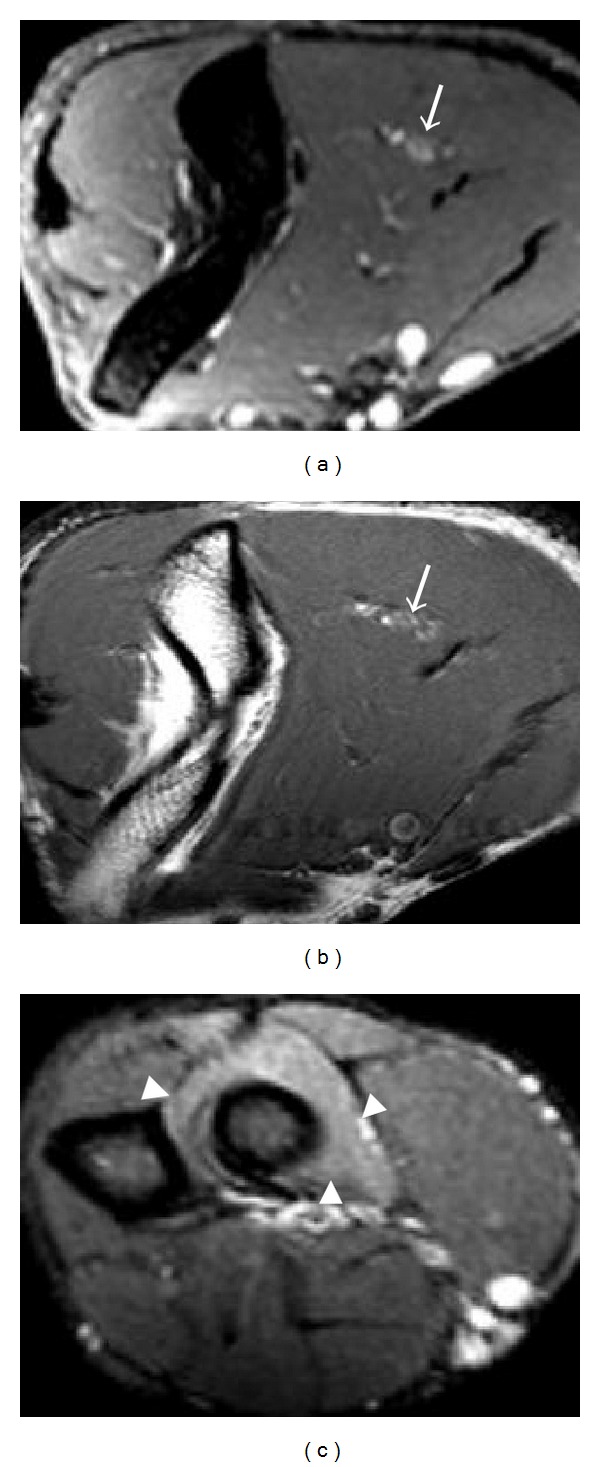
An 18-year-old patient with clinical and EMG evidence of PIN entrapment. Axial T2 fat-saturated (a) and T1-weighted (b) images at the level of right distal humerus show thickening and high T2 signal of the radial nerve (arrows). Supinator muscle edema (arrowheads) is present (c), axial T2 fat-saturated image.
